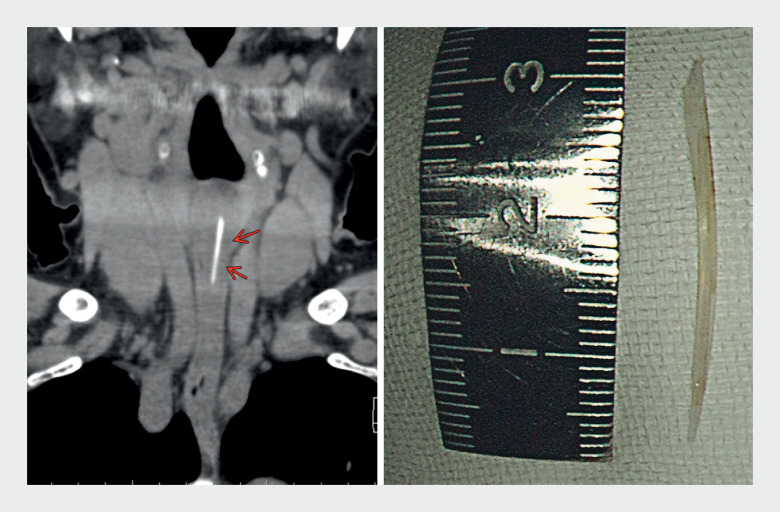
Photograph of the extracted fishbone (right), which is seen to be the same shape as on computed tomography image (left).
